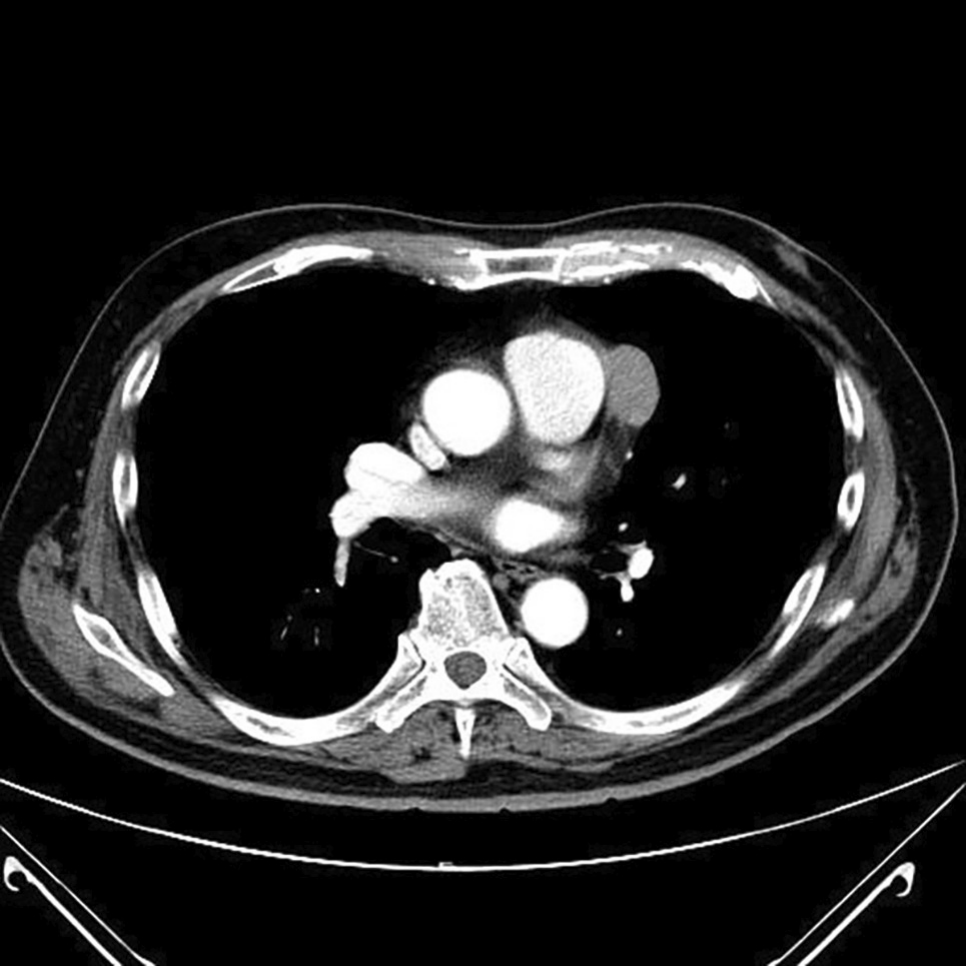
Axial contrast‐enhanced computed tomography (CT) image of the chest showing a 33‐mm well‐defined, homogeneous mass with partial calcification located in the left anterior mediastinum adjacent to the main pulmonary artery. The lesion was initially suspected to be a thymoma.
